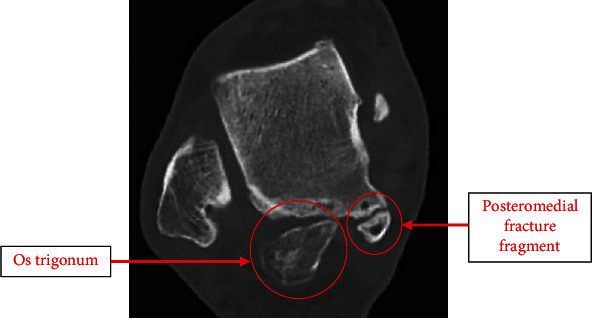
Axial CT slice demonstrating lateral os trigonum and posteromedial talus fracture fragment nonunion.
